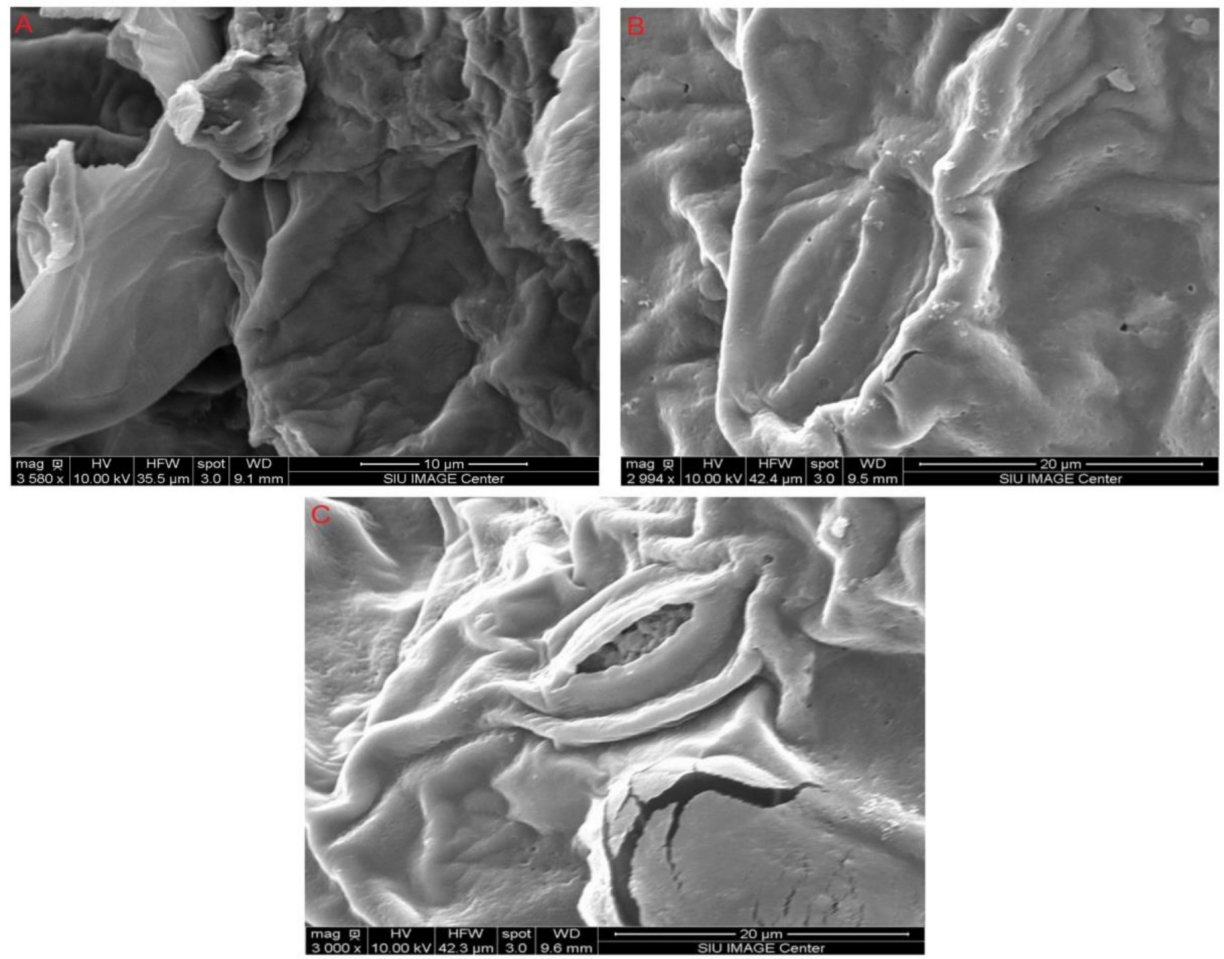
SEM images peach samples. Panel (A) a non-treated (B) a sample after solvent extraction. Panel (C) a sample after ultrasonic treatment sample.
